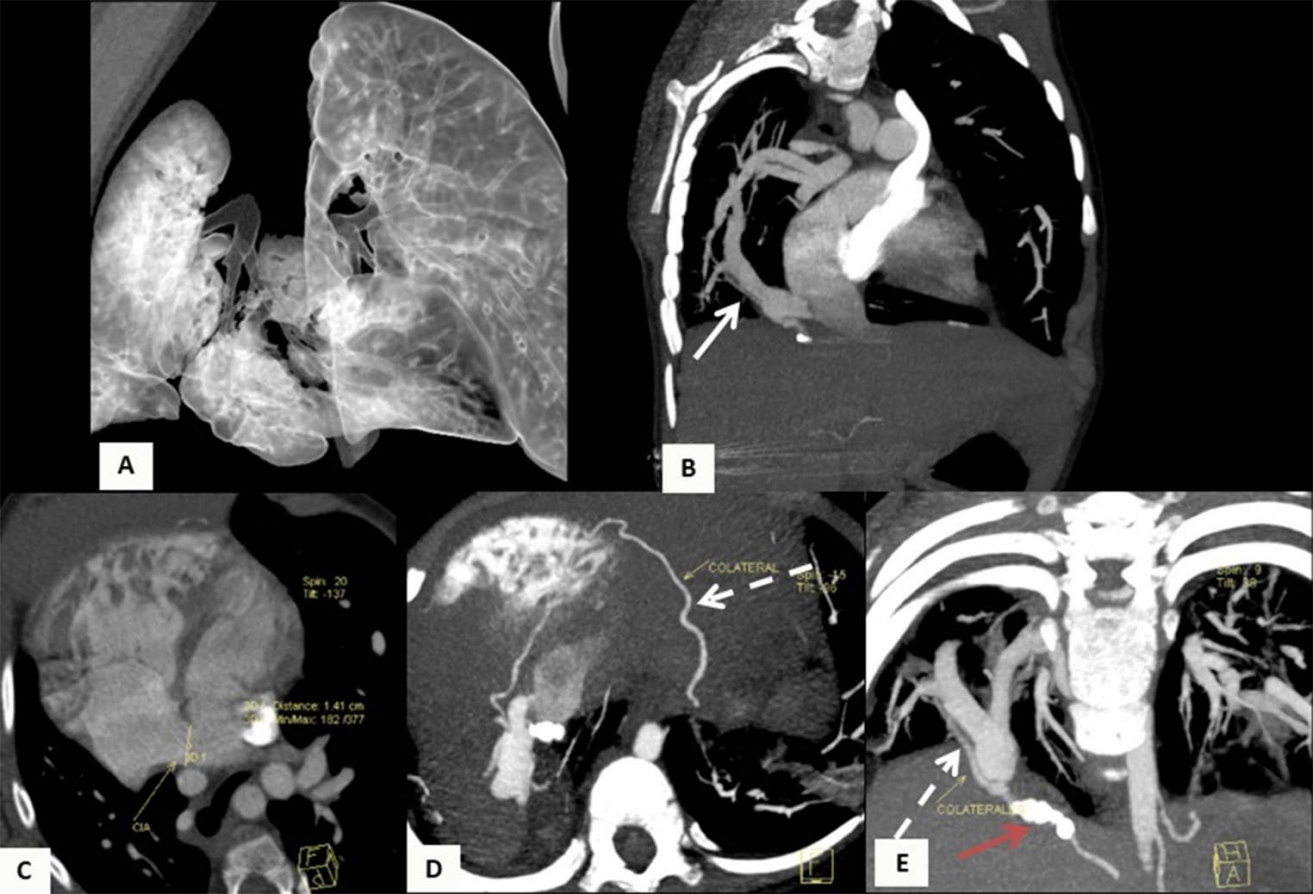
3-year-old male patient with Scimitar syndrome. (A) 3D rendered lungs image shows decreased volume in the right lung. (B-E) Chest Angio-CT with multiplanar reconstructions. (B) Maximum intensity projection (MIP) oblique-coronal reconstruction shows anomalous right pulmonary venous drainage into the inferior vena cava (white arrow). (C) Axial reconstruction shows a large atrial septal defect (ASD) as part of the Scimitar syndrome. (D) MIP oblique-axial maximum intensity projection and (E) MIP oblique-coronal reconstruction, show aortopulmonary collateral circulation (dashed arrows) arising from diaphragmatic arteries directed towards the connection point between the anomalous venous drainage and the inferior vena cava, with post-embolization changes (red arrow)
